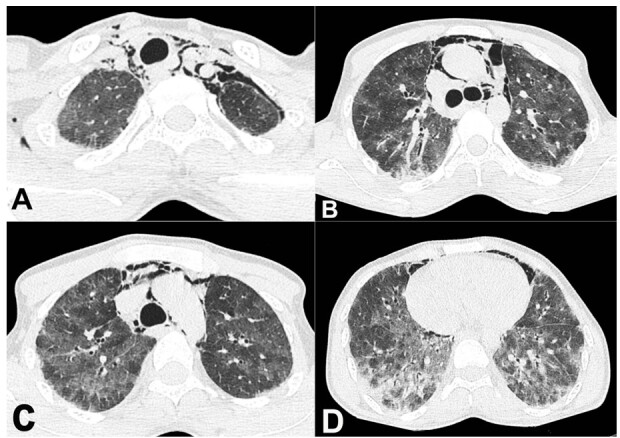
Thoracic HRCT - A, B, C, and D axial plane showing pneumomediastinum, subcutaneous emphysema, bilateral patchy areas of GGOs with interspersed septal thickening, and basal, subpleural, patchy consolidation. Also noted is a mild left-sided pneumothorax in A.
